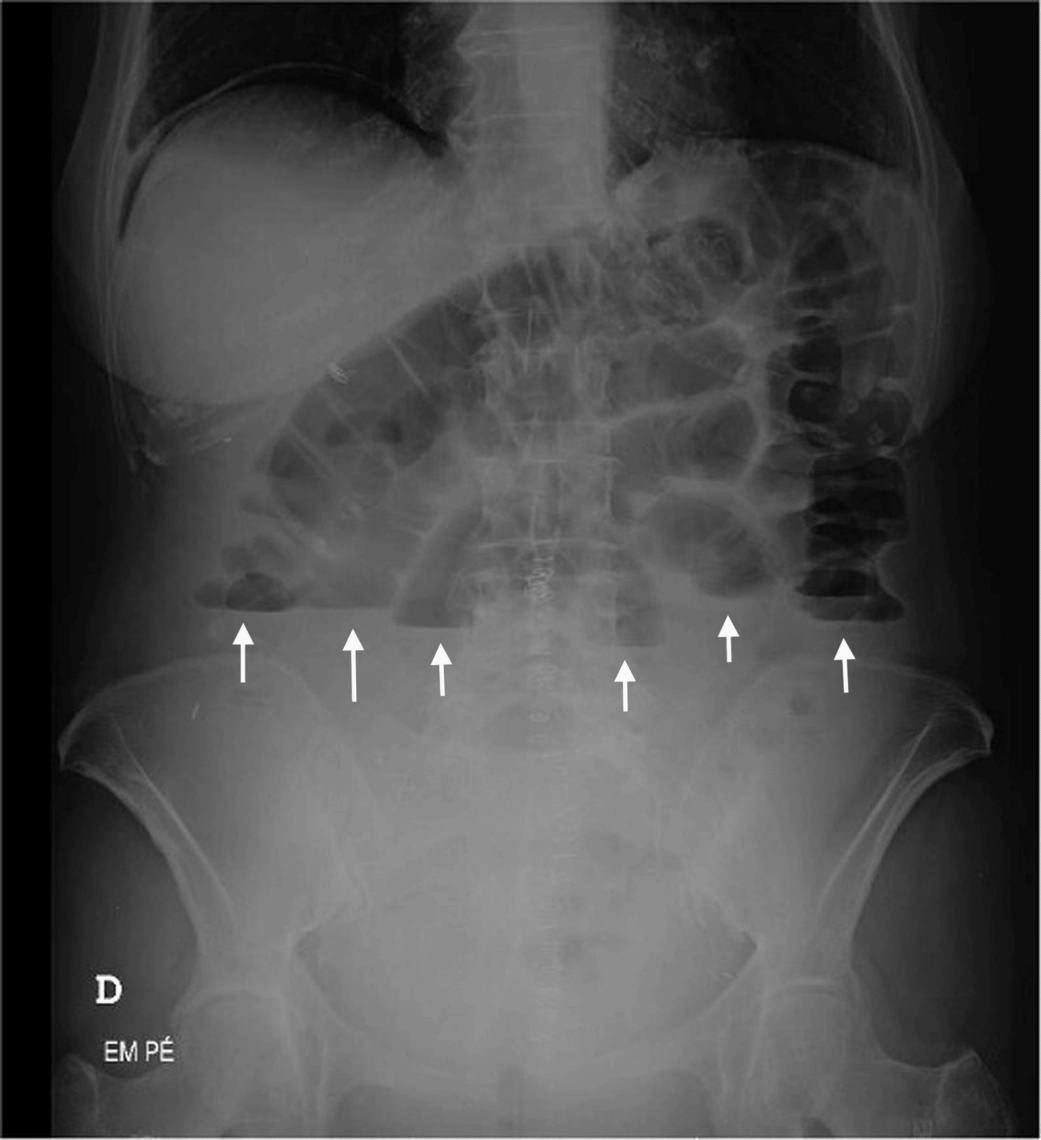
Upright abdominal X-ray demonstrating small-bowel air-fluid levels (arrows)
